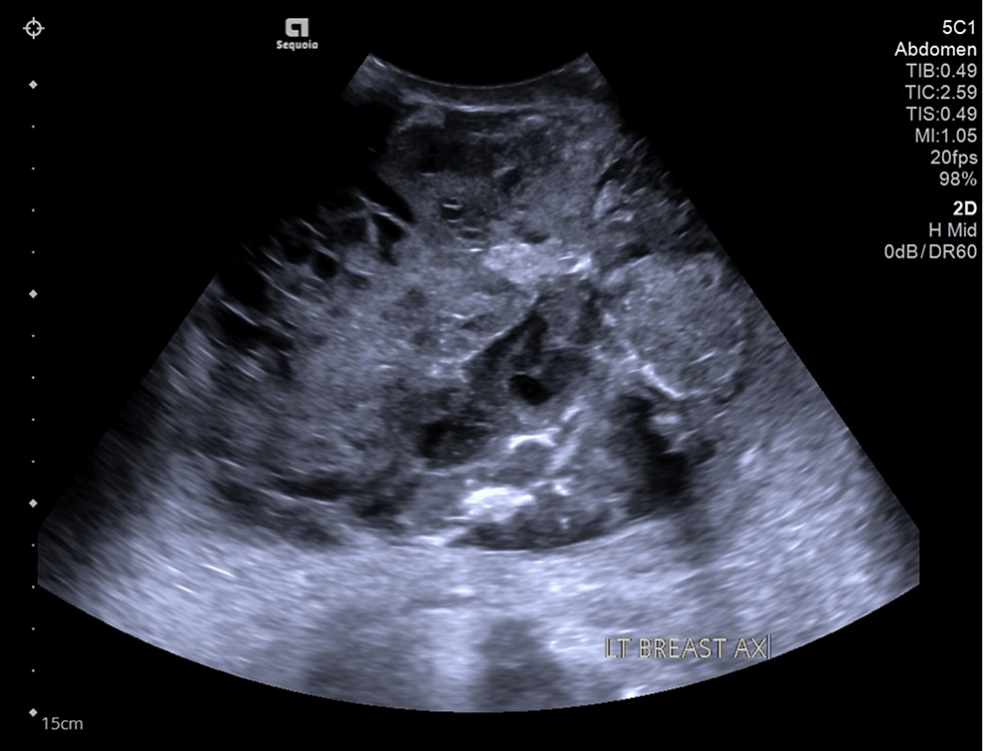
Ultrasound of the left breast showing a large heterogeneous solid mass with cystic components within.
